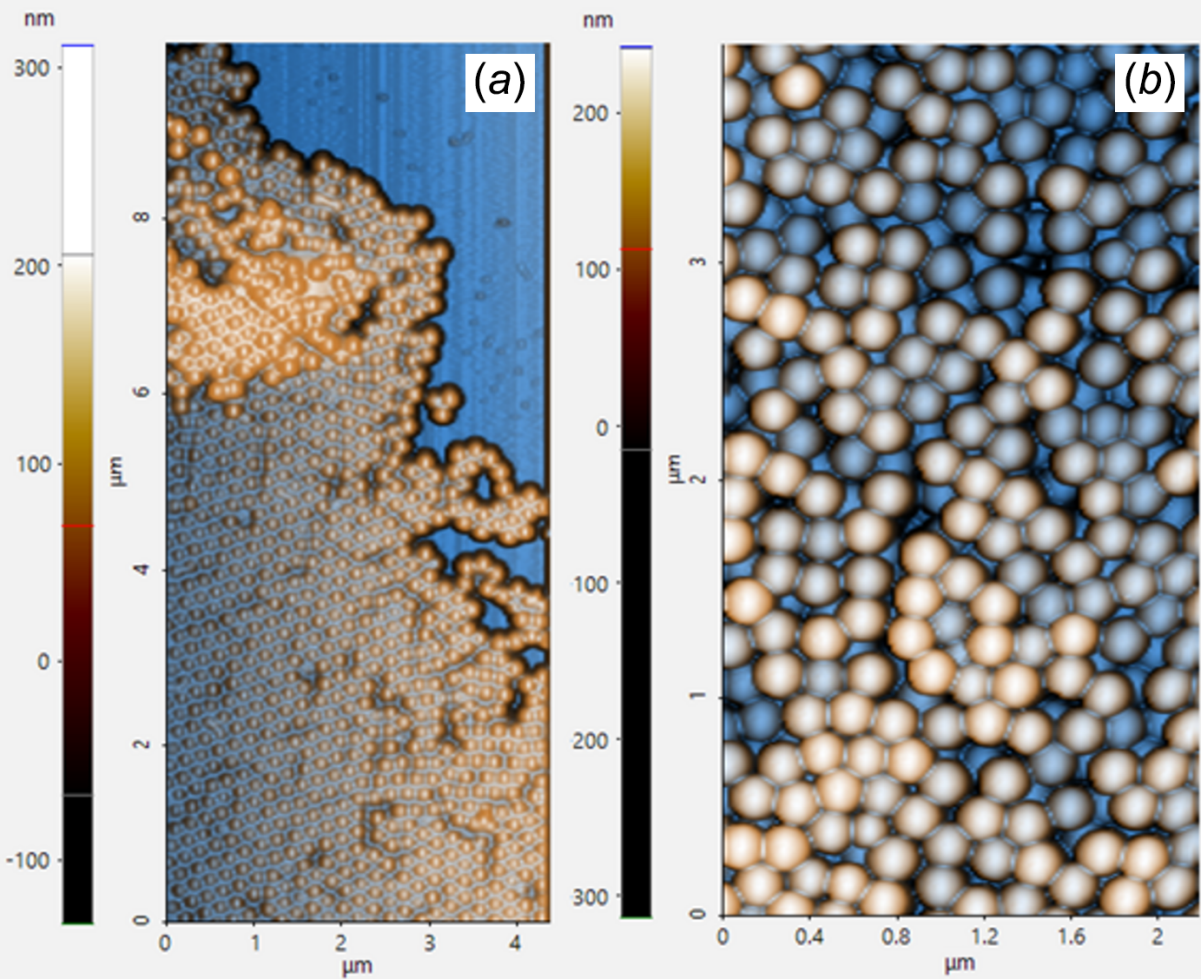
AFM 3D image of the PPS colloid agglomerate, transferred from the air/water interface after the X-ray experiment.
